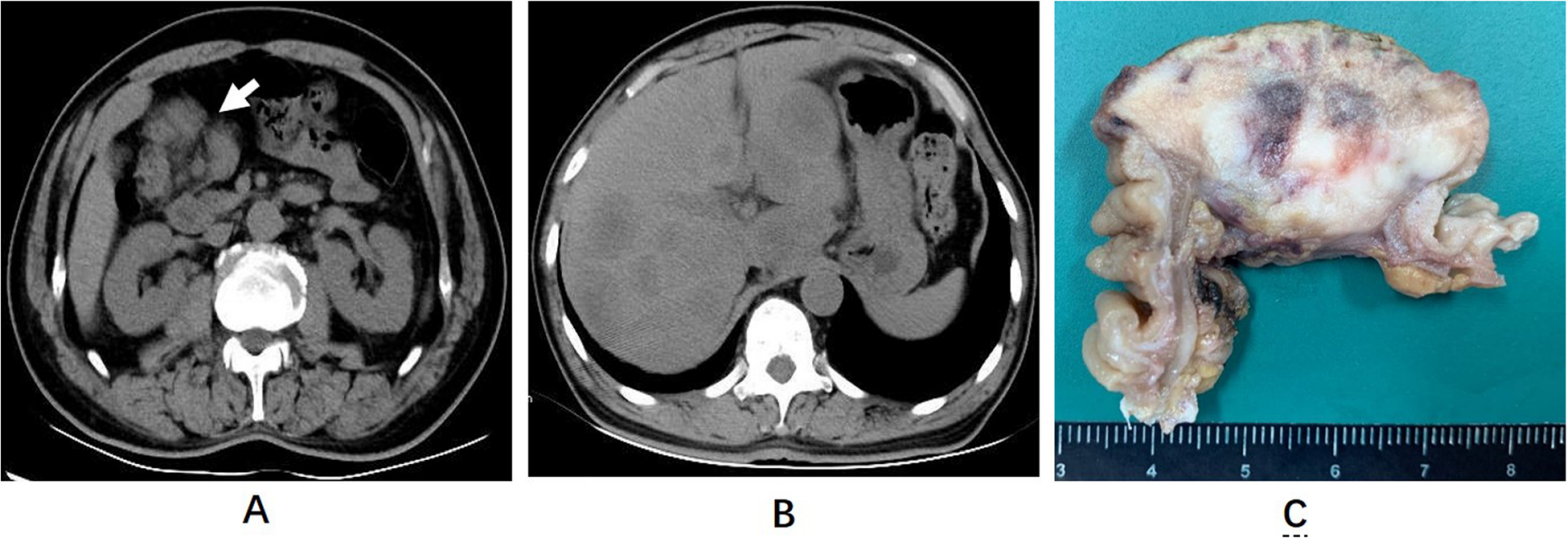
Axial CT scan at the level of the kidneys (a) and liver (b) demonstrates a mass (white arrow) within the hepatic flexure of the colon and multiple liver masses, respectively. The cut surface (c) shows the tumor has penetrated through the muscle wall of the colon, exhibiting fine texture with central hemorrhagic necrosis
